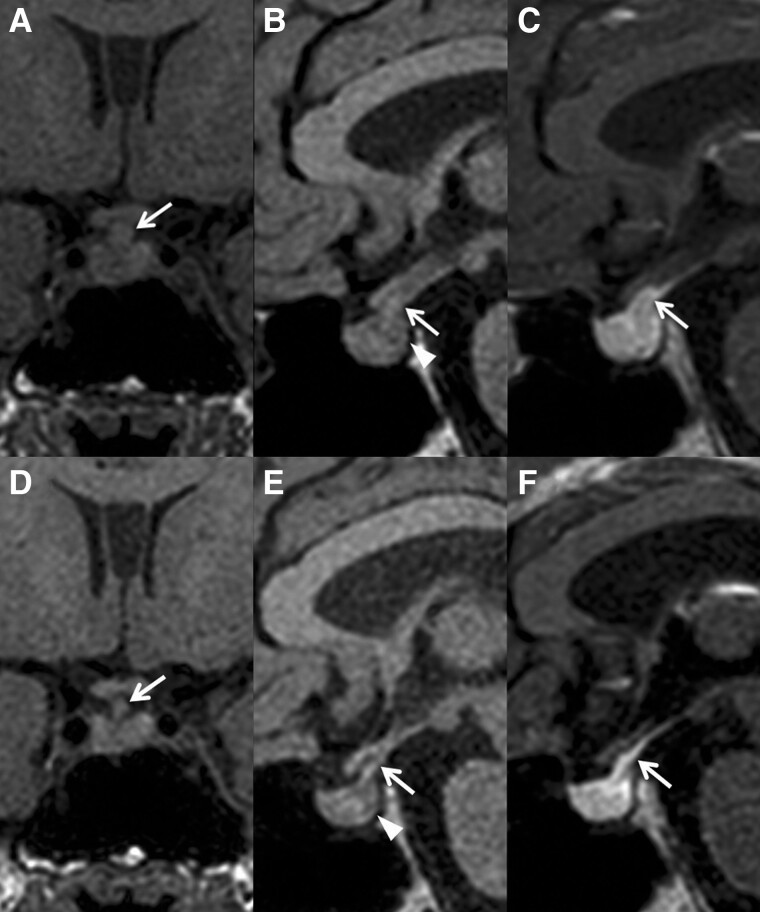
Magnetic resonance imaging of hypothalamic-pituitary regions. (A-C) Thickening of the pituitary stalk (arrows) and the absence of the posterior pituitary bright spot (arrowhead) at initial presentation (A, B: noncontrast T1-weighted; C: contrast-enhanced). (D-F) Regression of the pituitary stalk thickening (arrows) and the persistent lack of the posterior pituitary bright spot (arrowhead) 1 year after presentation (D, E: noncontrast T1-weighted; F: contrast-enhanced).
